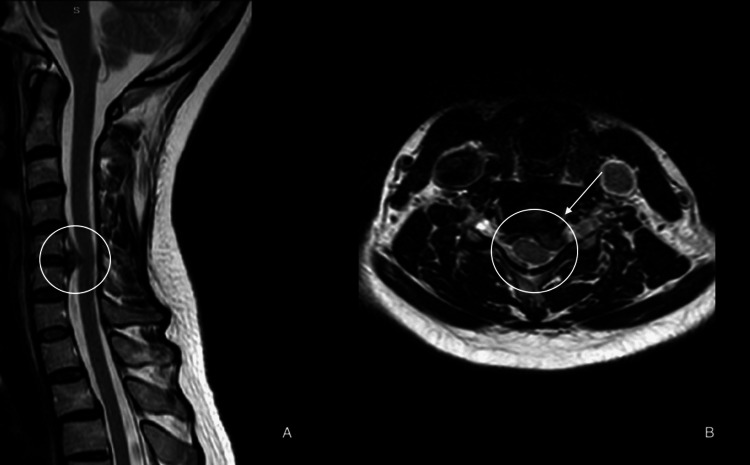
(A) Sagittal and (B) axial T2-weighted MRI of the cervical spine showing a large C5-C6 foraminal/paracentral disc protrusion with canal stenosis. MRI, magnetic resonance imaging
